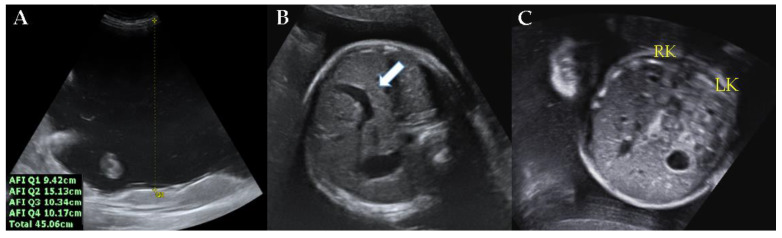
Ultrasonographic findings at 31 weeks of gestation. (A) Amniotic fluid index was 45.06 cm. (B) Persistent right umbilical vein (arrow) was observed. (C) There were no specific findings in either kidney of the fetus. AFI, amniotic fluid index; LK, left kidney; RK, right kidney.
